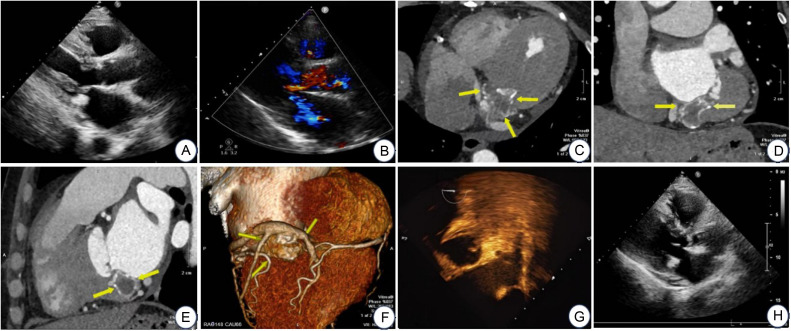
Fig. (A–B) Transthoracic echocardiography (TTE). Fig. A (parasternal long-axis view) shows a well-defined hyperechoic mass along the posterior mitral annulus. Fig. B (color Doppler) demonstrates no internal blood flow, suggesting a nonvascular lesion. Fig. (C–F) Coronary computed tomography angiography (CCTA). Figs. C–E (axial, coronal, and sagittal MIP images) demonstrate a ring-shaped heterogeneous lesion along the posterior mitral annulus (yellow arrows) with eggshell-like calcification, characterized by a hyperdense rim and hypodense center, clearly separated from the left atrial and ventricular cavities. Fig. F (VR image) shows circumferential mitral annular calcification and its spatial relationship with the coronary arteries. Fig. (G–H) Transesophageal echocardiography (TEE). No spontaneous echo contrast or thrombus is detected in the left atrium or left atrial appendage.
